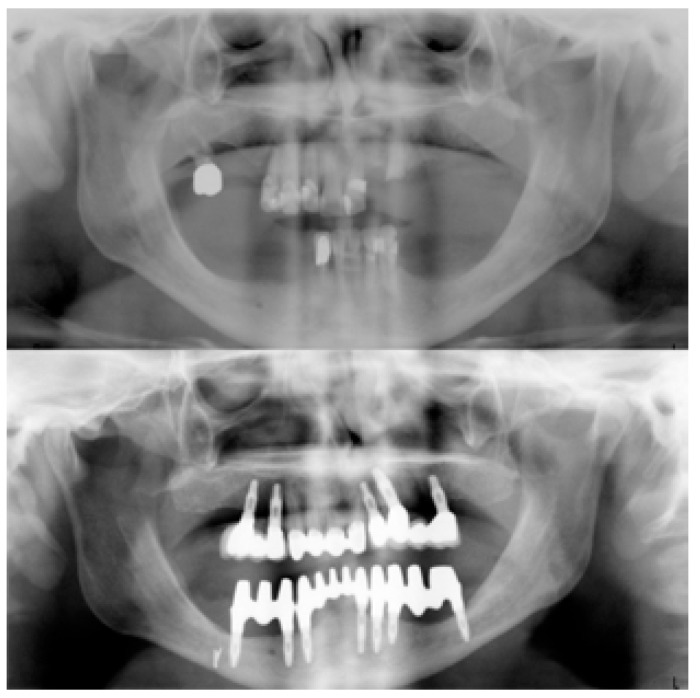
Preoperative and postoperative orthopantomographies: The maxilla was rehabilitated using both tooth and implant supported fixed partial dentures; and the lower jaw using the Toronto Prosthesis.
